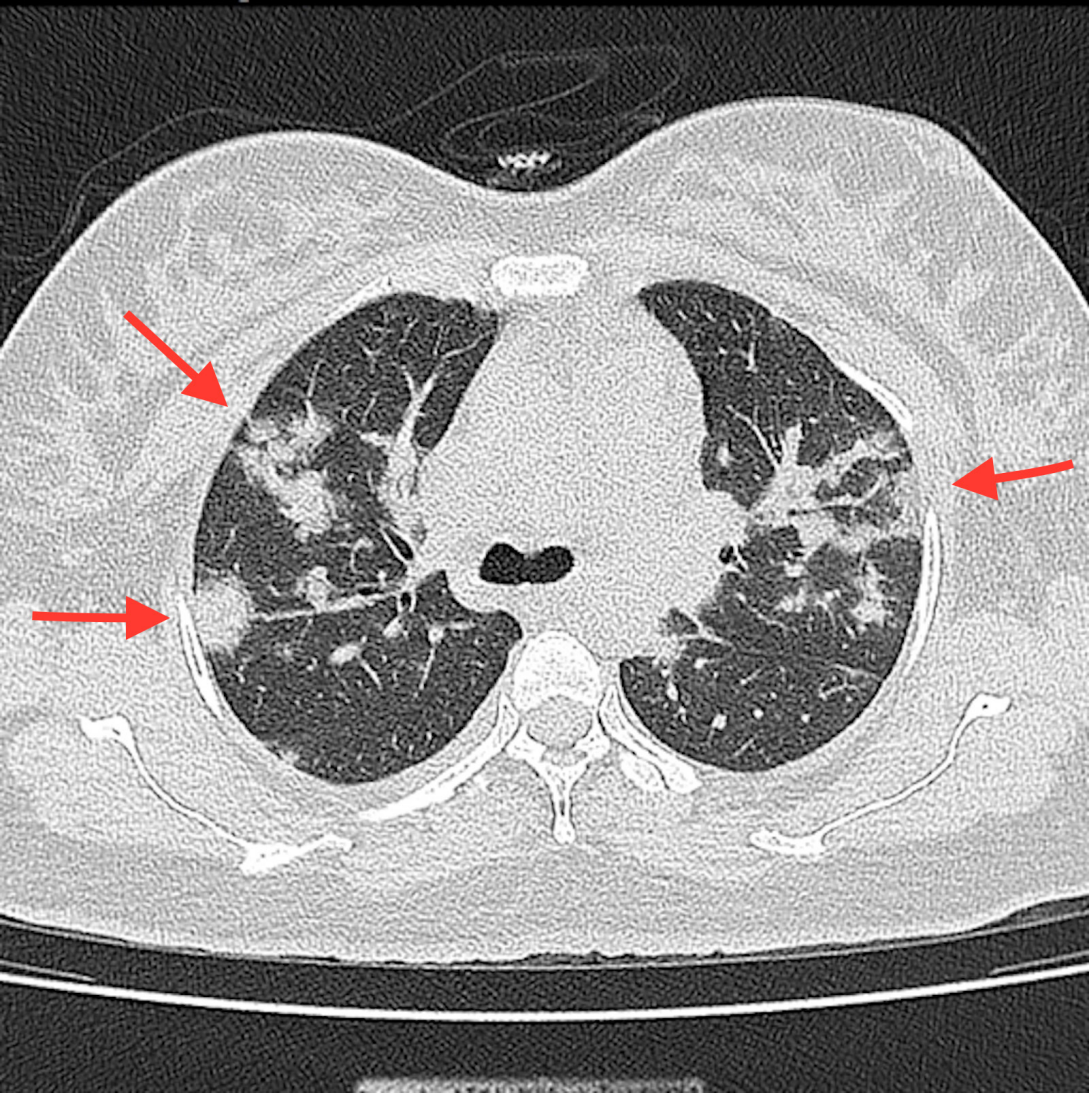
Multifocal ground‐glass opacities in low‐dose high‐resolution chest computed tomography (HRCT)
